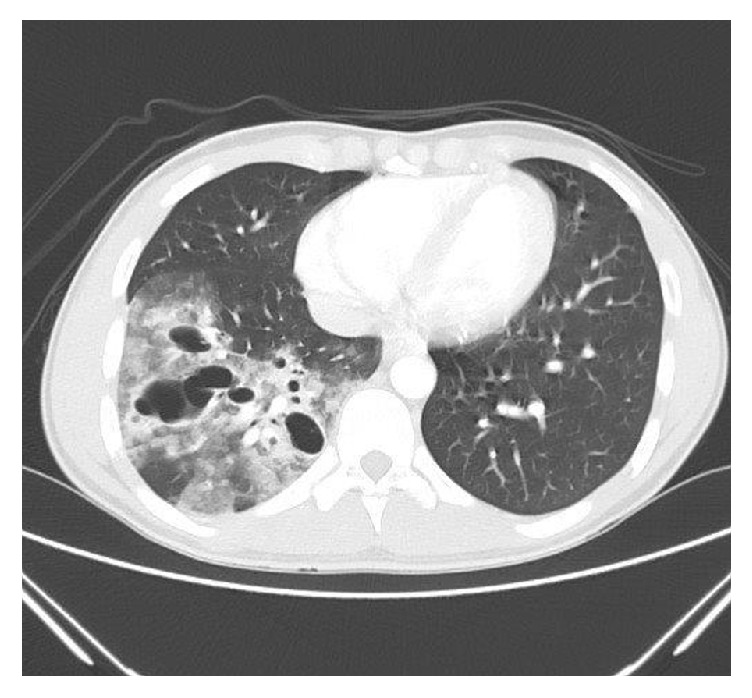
CT scan of the chest showing thick-walled multicystic lesions with patchy air space opacities.
